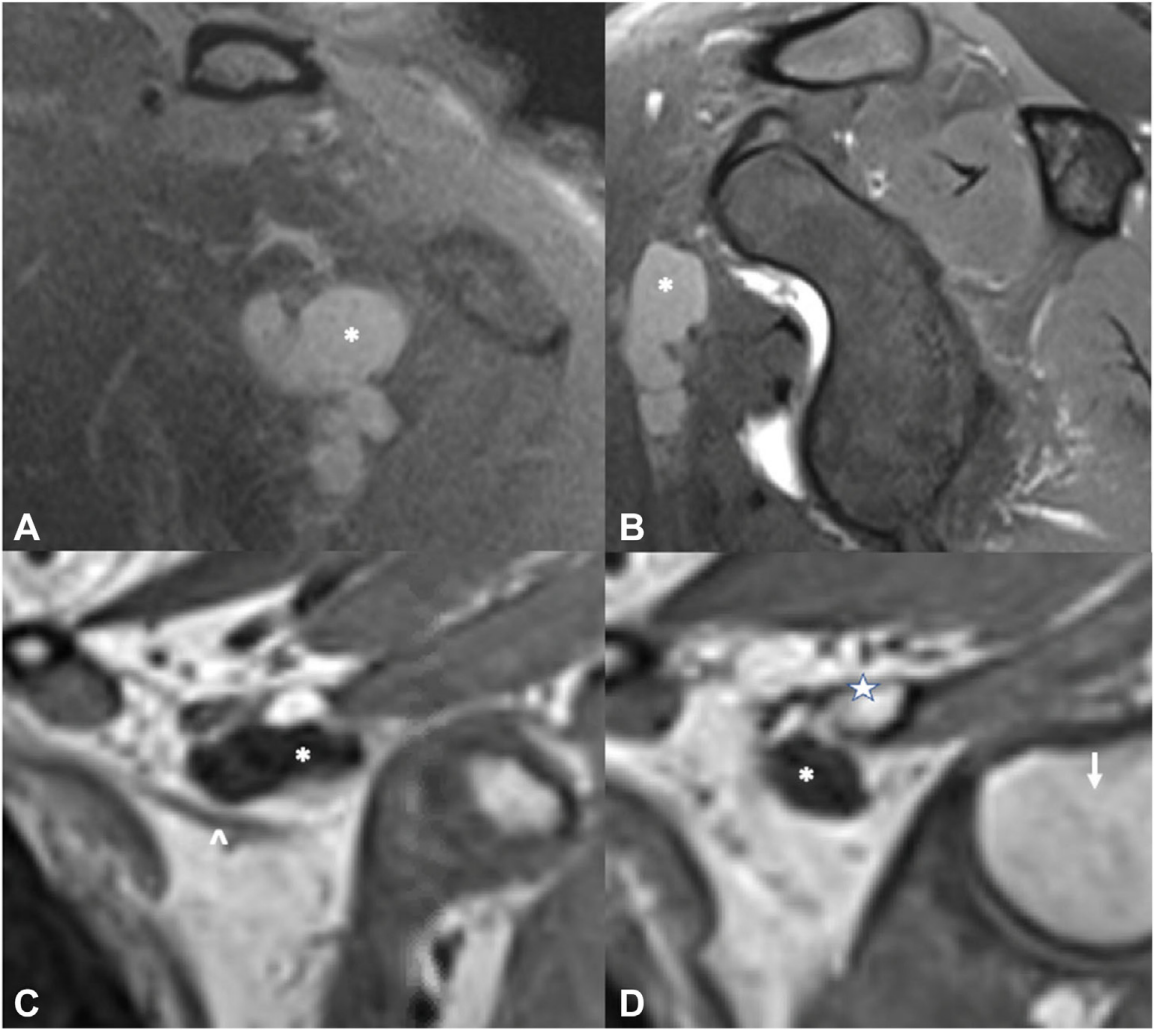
Large subcoracoid cyst noted on the magnetic resonance imaging of the left shoulder and brachial plexus in a patient with vague, deep pain around the anterior shoulder and upper chest, worse with repetitive activity. A Coronal view; cyst marked with white ∗. B Sagittal view; cyst marked with white ∗. C Anterior coronal slice; cyst marked with white ∗, plexus marked with white ˆ. D Posterior coronal slice; cyst marked with white ∗, coracoid marked with white star, and humeral head marked with white downward arrow. The patient was treated with arthroscopic PM release, cyst decompression, and brachial plexus neurolysis, with resolution of her symptoms and return to activity.
